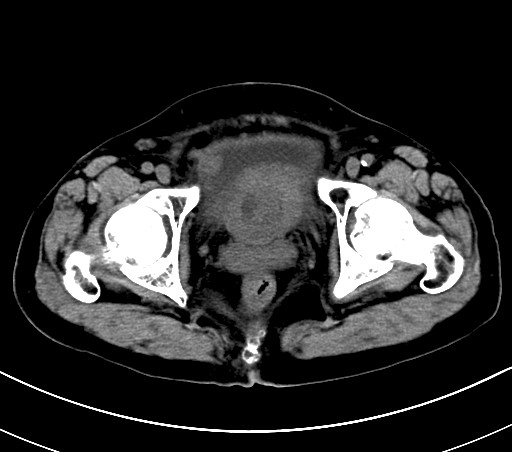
Two months after second TUR, CT showed that the tumor recurrence again at the anterior wall and the neck, invading urethra downward.
